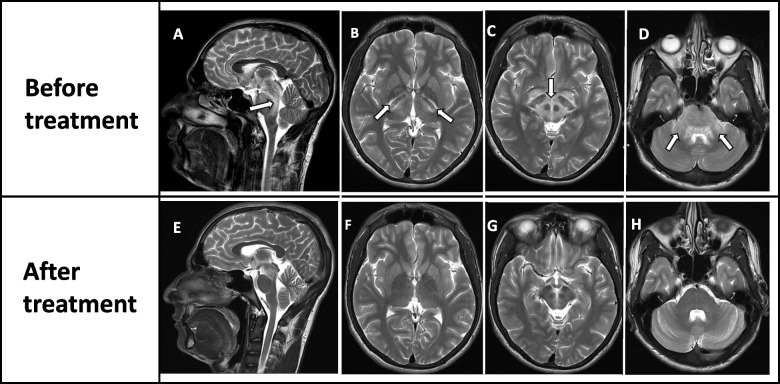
T2W MRI images of the brain before and after haemodialysis. The hyperintensities seen in the mid brain and pons (sagittal) (A), internal capsule (B), midbrain (except substantia nigra, red nucleus, and superior colliculus) (C) and middle cerebellar peduncles (D) are markedly reduced after haemodialysis (E to H)
